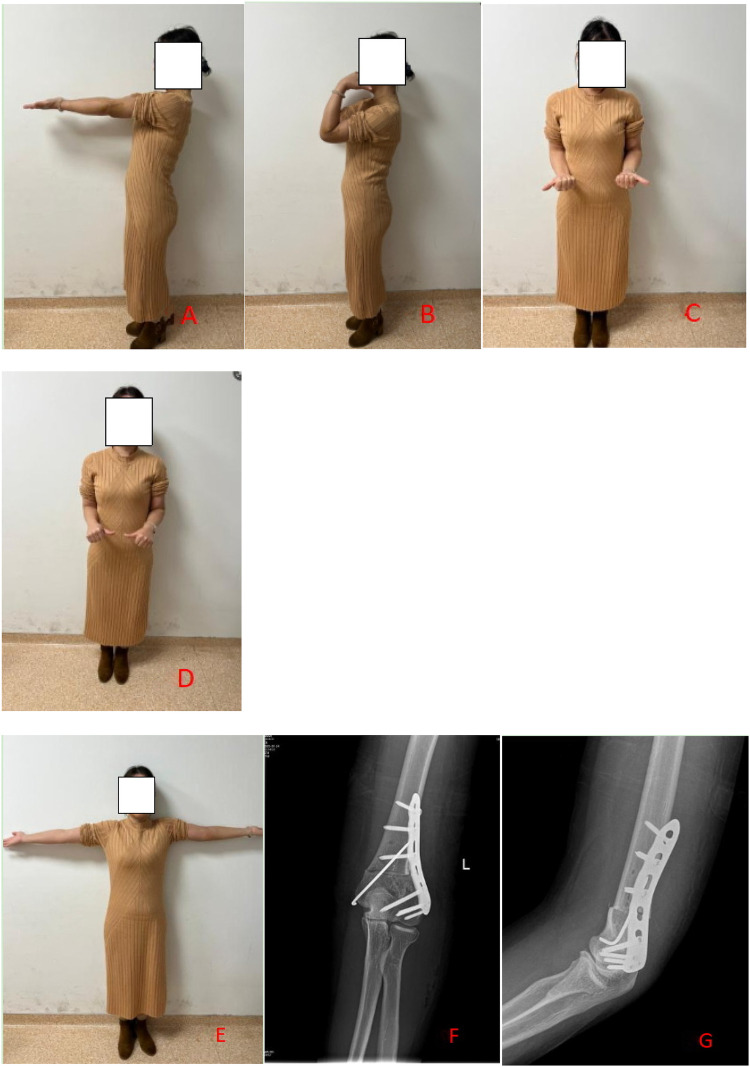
(A–D) the left elbow showed excellent function and same with normal right side two weeks postoperation. (E–G) Postoperative photograph and radiographs showed cubitus varus deformity of left elbow has been accurate corrected.
